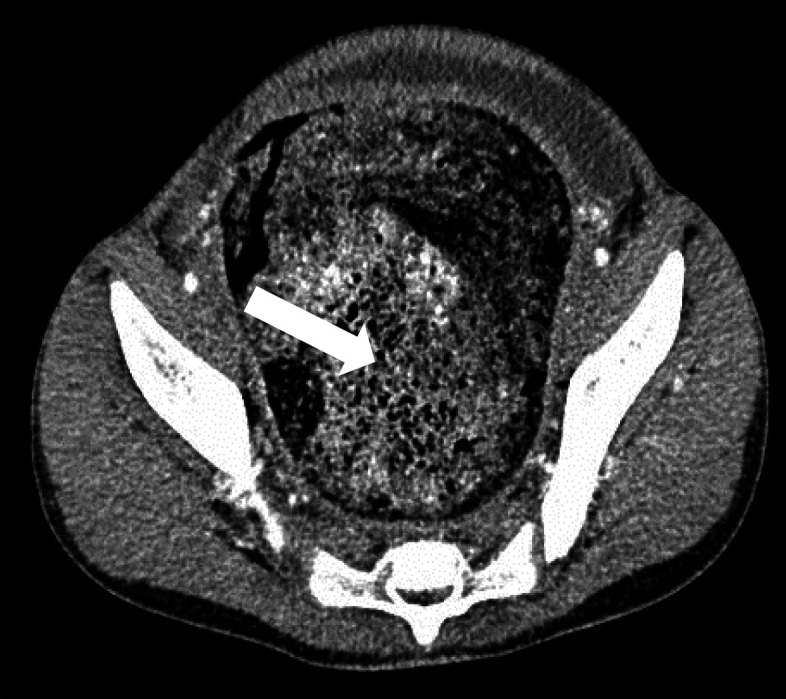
Contrast-enhanced axial CT section showing well-formed, large fecal ball in the dilated rectosigmoid colon
